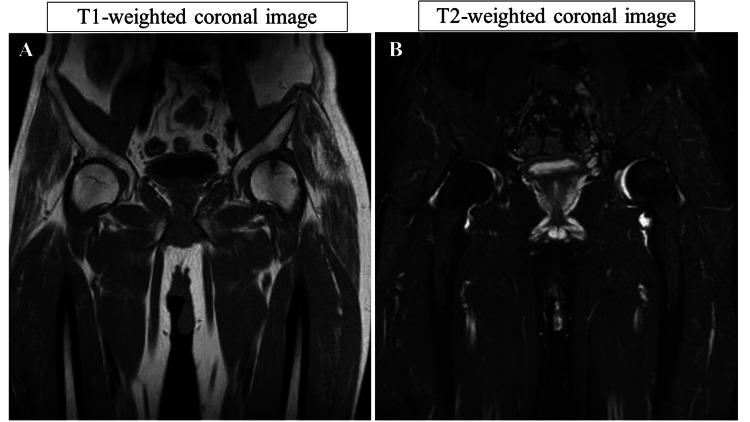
Postoperative hip imaging (MRI) (A) Postoperative hip MRI (T1-weighted coronal image). (B) Postoperative hip MRI (STIR image). Postoperative hip imaging (MRI) reveals the resolution of synovial bursitis. MRI: magnetic resonance imaging, STIR: short tau inversion recovery
